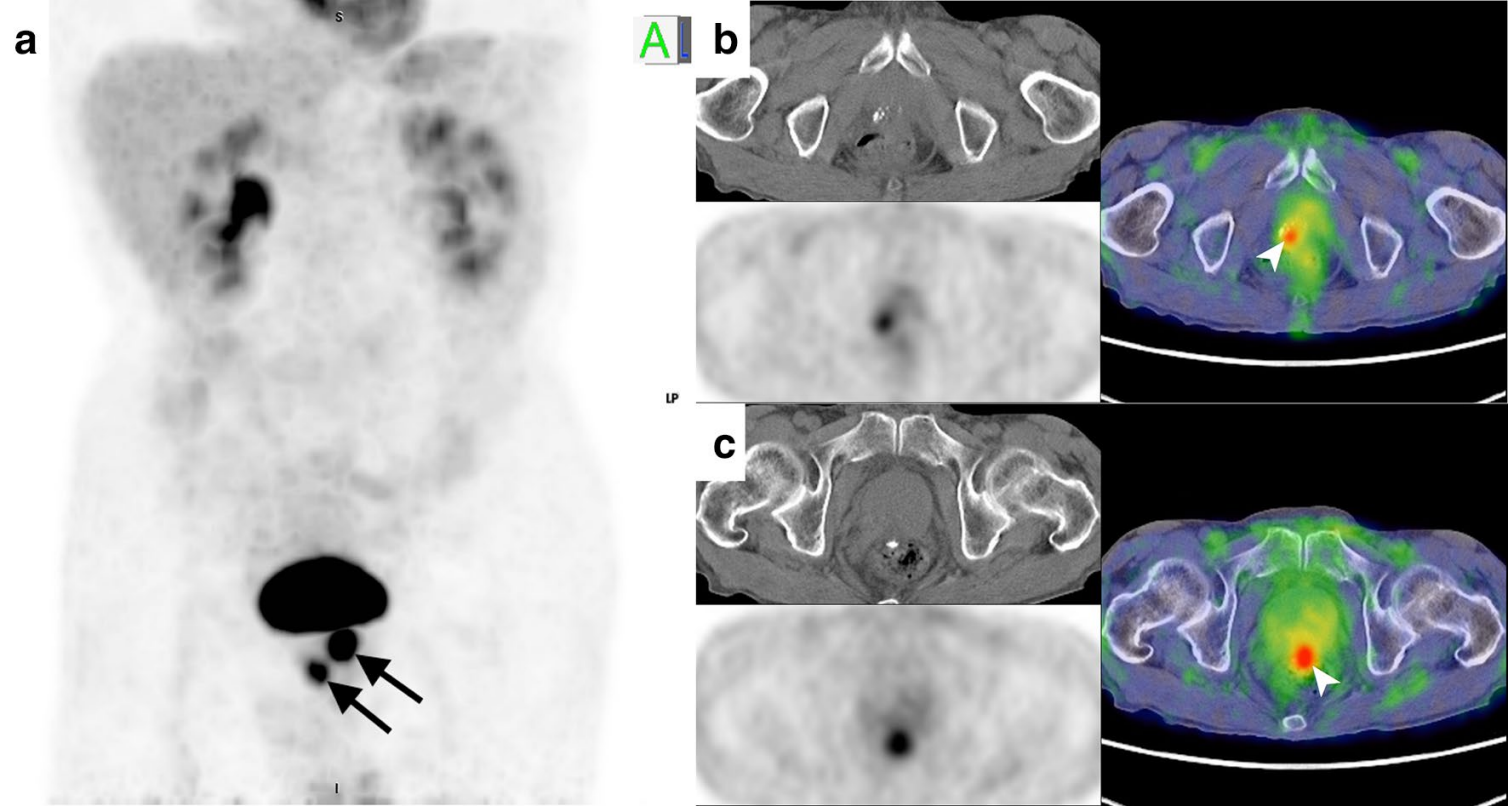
FDG-PET/CT. FDG-PET MIP image (a) showed two incidental foci of FDG uptake in the prostate gland (→). On PET/CT, the both lesions at the apex (b) and the midgland (c) included calcification ()
